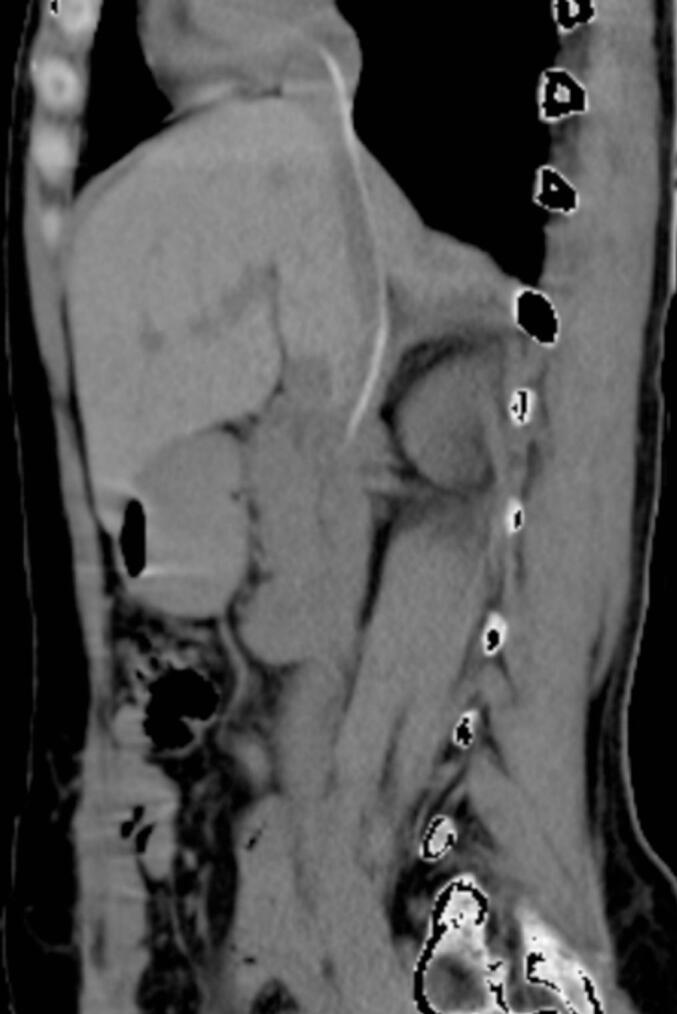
CT scan showing radio-dense tubular foreign body in the IVC.
